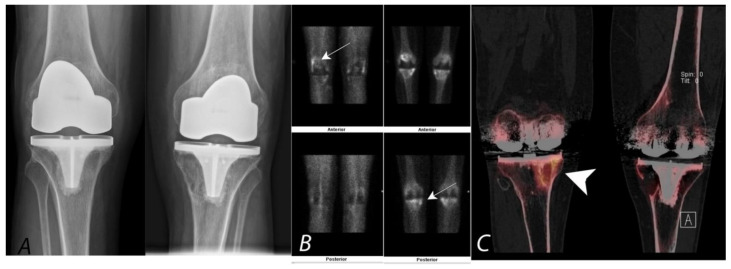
Bilateral anterior AP radiographs, anterior and posterior planar blood pool and delayed mineral phase images and coronal SPECT/CT images of a Tc-99m MDP bone scan in a 65-year-old male with bilateral painful total knee protheses. Plain radiographs (A) are non-specific (and unchanged over several years) without defined peri-prosthetic lucency. Blood pool images and delayed images (B,C) demonstrate some hyperemia in the joint capsule and serpiginous areas of proximal tibial uptake (white arrows). Clarity is achieved by the SPECT/CT (coronal images, (C)), which demonstrates uptake associated with large, well-corticated areas of bone loss consistent with particle disease (white arrowhead). A similar well corticated region of lucency is also present in the medial aspect of the left proximal tibia.
